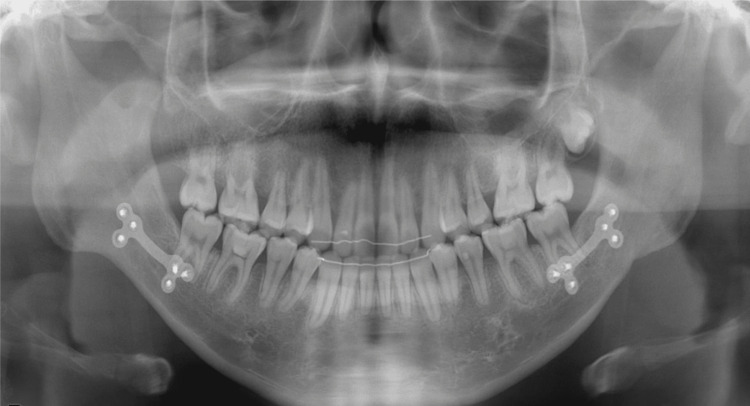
Post-treatment evaluation. Panoramic Radiograph.
